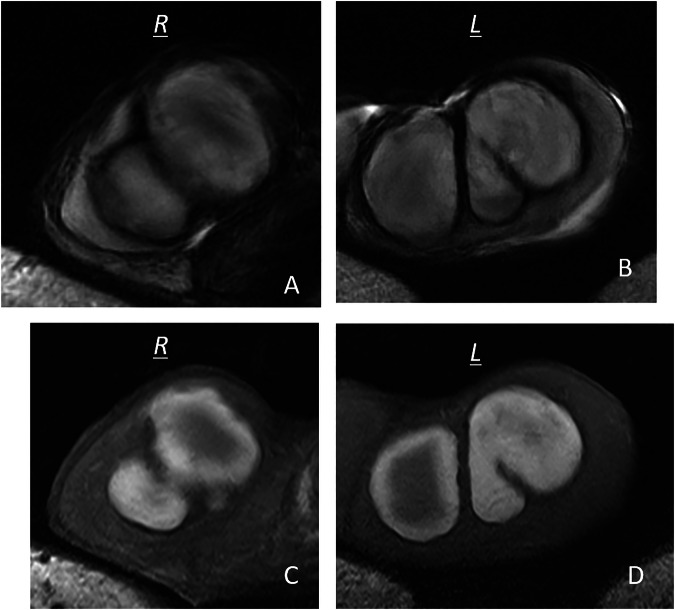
Bilateral spontaneous hematomas in a patient with liver cirrhosis, MRI findings (same patient as in Fig. 3). Typical hematomas as previously described, with rims in hypo signal on T2-weighted images (A, B) and exhibiting a hypersignal including an internal core in isosignal on T1-weighted images (C, D). Additional sequences could not be acquired due to patient discomfort
